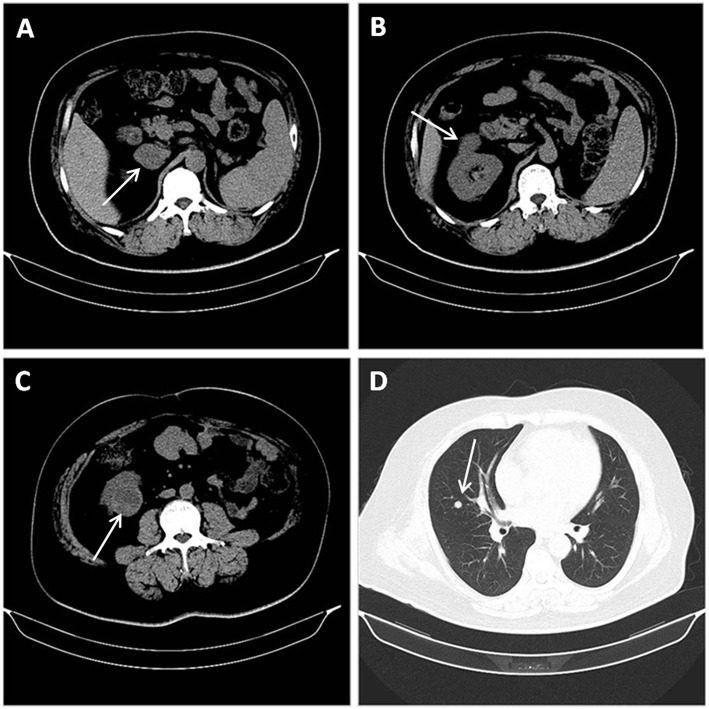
Abdominal and pelvic non-contrast CT revealing a 36 × 31-mm, well-demarcated, ellipsoid and hypodense mass with a CT value of about 27 Hounsfield units (HU) in the external branch of the right adrenal region. (A) Hypodense lesions with a diameter of 15 mm and 39 mm discovered in the upper (B), and lower (C) poles of the right kidney, respectively. Non-contract CT of the chest suggesting lung metastasis: multiple nodules in both lungs, the largest one being located in middle lobe of the right lung (D).
